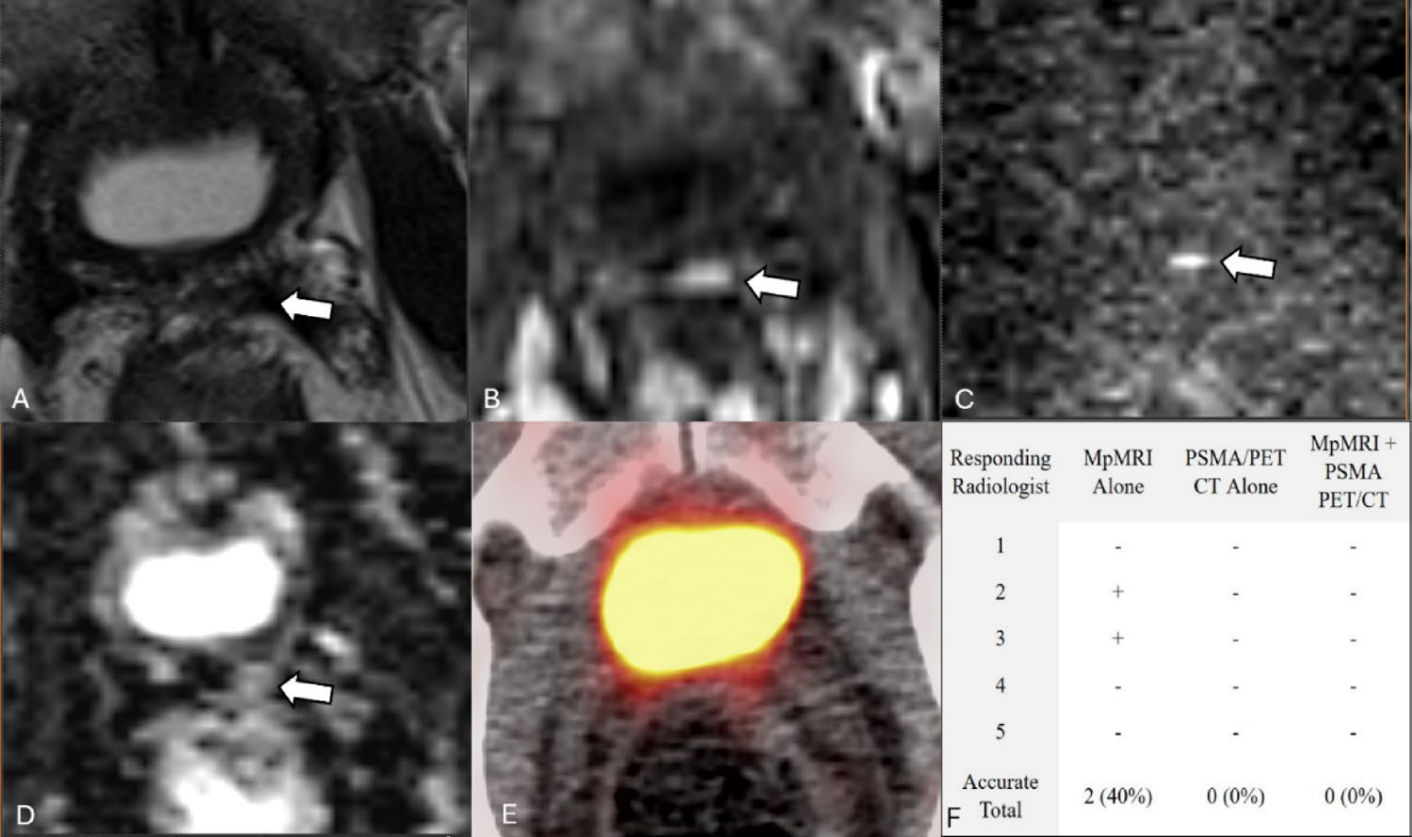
78-year-old patient with a left prostate bed lesion with early enhancement and restricted diffusion on mpMRI but no focal uptake on PSMA PET/CT. This case depicts a 78-year-old status-post prostatectomy indicated for a primary adenocarcinoma with a Gleason score of 4 + 4 = 8 with a T3b pathological stage. This patient also received salvage radiation treatment before imaging. His PSA was measured to be 2.4 ng/mL. MpMRI (A T2, B Arterial enhancement T1, C DWI, D ADC) shows a 5–10 mm T2 dark lesion with early enhancement and restricted diffusion in the left prostate bed (white arrow). Gallium-Ga 68 PSMA-11 PET/CT (E) shows no focal uptake in this region. MpMRI and PSMA PET/CT were performed 3 days apart. Radiologist performance worsened with both imaging modalities in conjunction compared to mpMRI alone (F)
